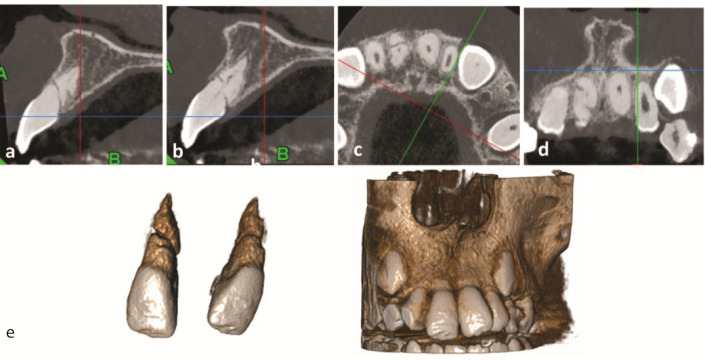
Ultra-low-dose CBCT section;
